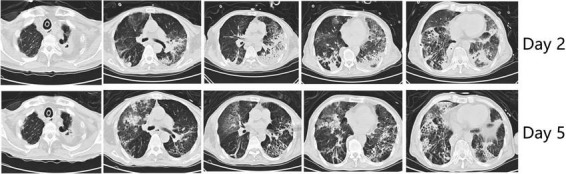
CT images of the lung. Upper of the picture presents CT images of patients at Day 2 and Lower reflects Day 5.
